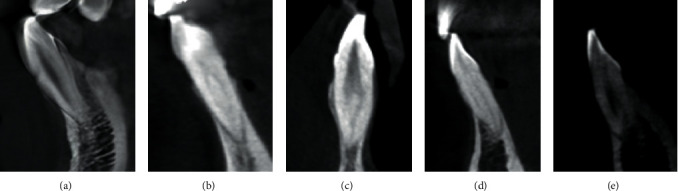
Sagittal CBCT sections of mandibular incisors show (a) one canal with type I Vertucci; (b) two canals with type II Vertucci; (c) two canals with type III Vertucci; (d) two canals with type IV Vertucci; (e) two canals with type V Vertucci.
